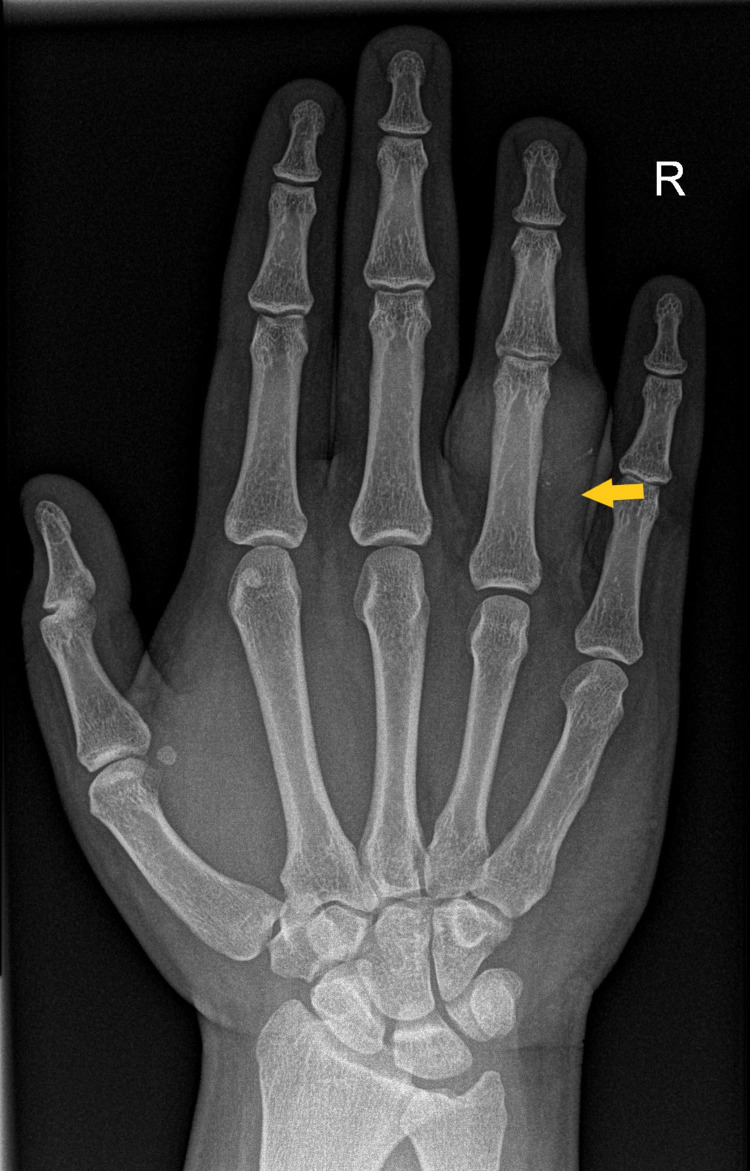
X-ray image showing soft tissue involvement of the affected ring finger (yellow arrow).
